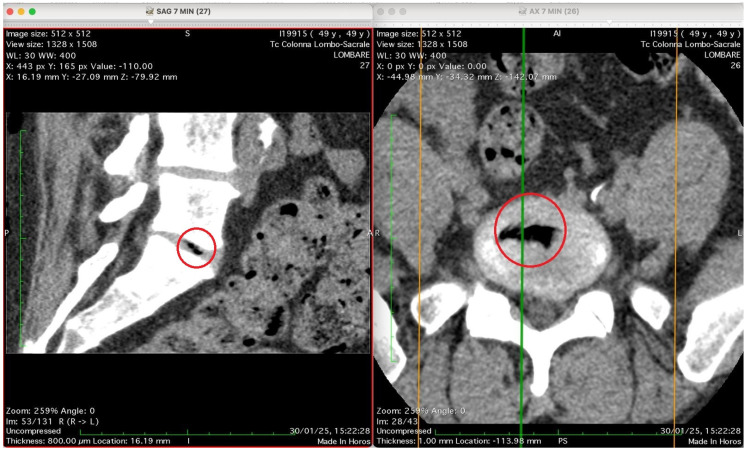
Sagittal (left) and axial (right) CT images of the lumbosacral spine at 7 min post-injection, showing a well-defined intradiscal gas pocket (red circle), likely at L4–L5 or L5–S1. The gas appears as a sharply demarcated, low-attenuation area within the disc, consistent with diffusion through annular fissures following paravertebral oxygen–ozone injection or vacuum phenomenon from degeneration. No extension into epidural or paravertebral tissues is observed. Neural structures and vertebral endplates remain intact. Technical details: 512 × 512 matrix, 0.8 mm slice thickness, WL/W: 30/400, scan date: 30 January 2025.
